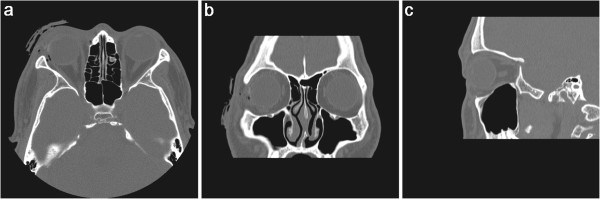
Unenhanced computed tomography findings of patient. a-b, Axial and coronal view showed accumulation of air near lateral rectus muscle insertion and swelling of preseptal soft tissues in right eye. a-c, But computed tomography images did not reveal radiopaque foreign body within the right globe.
